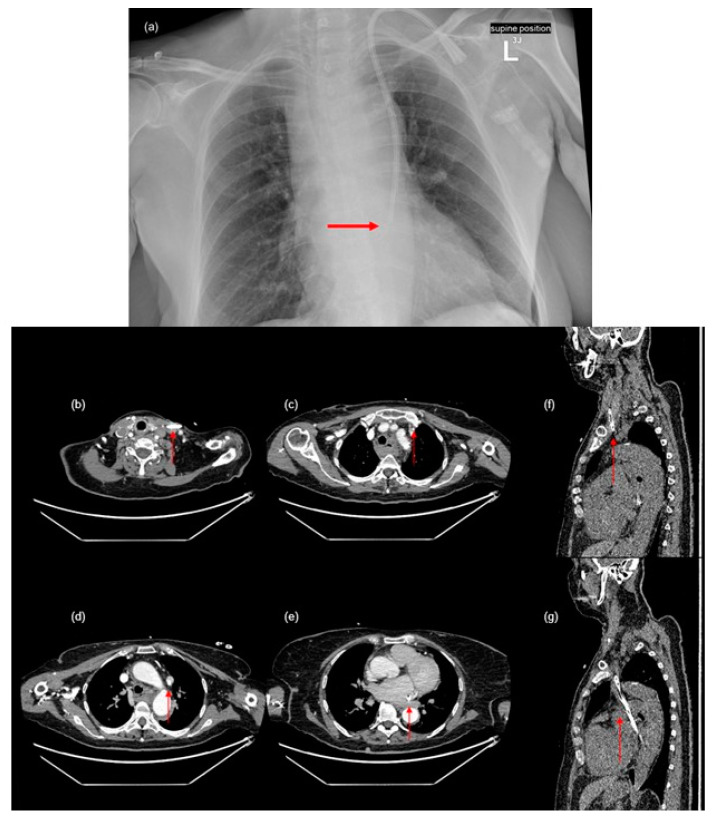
Posterior to anterior chest X-ray with a central venous catheter inserted into the persistent left superior vena cava (arrow in (a)). Persistent left superior vena cava in computed tomography ((b–g)—arrows indicate the path that the catheter passed).
